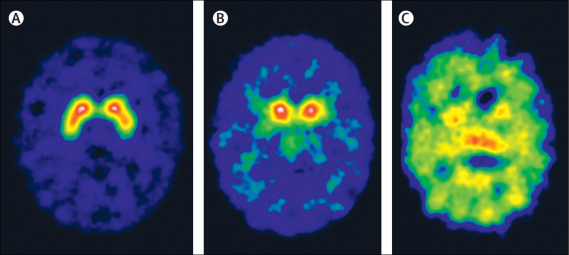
Nuclear brain imaging with single photon emission CT DaTSCAN in a control patient (A), a patient with juvenile parkinsonism of unknown aetiology (B), and patient 3 (C) Patients were age-matched. (A) Normal distribution of dopamine transporter sites. (B) Bilateral symmetrical loss of dopamine transporter sites from lentiform nuclei. (C) Complete loss of dopamine transporter sites in the basal nuclei, resulting in high background counts without any activity from basal nuclei.
